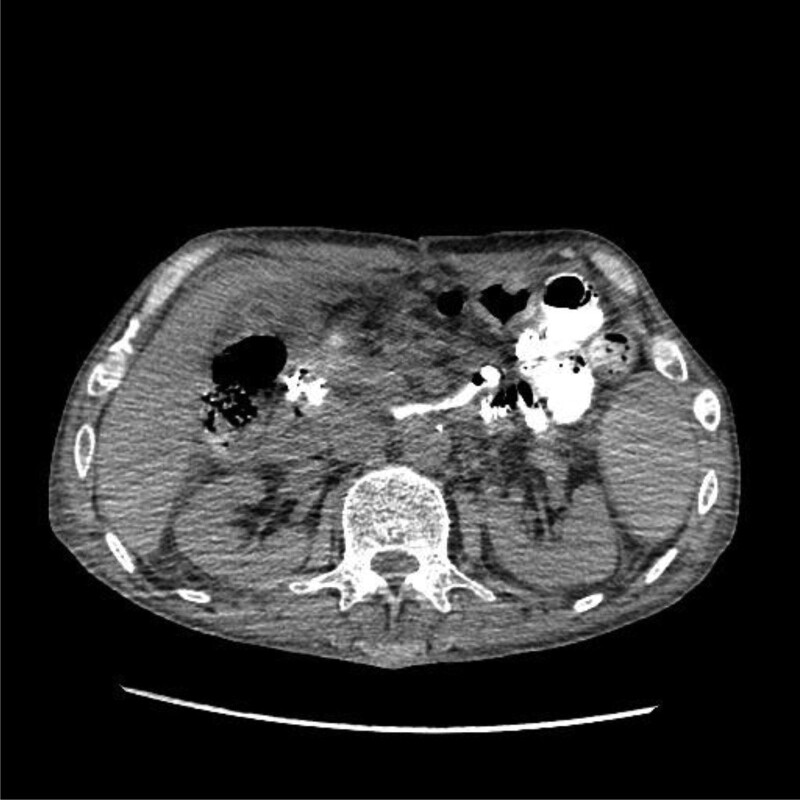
Abdominal computerized tomography (CT) examination was performed 15 minutes after oral contrast agent. Contrast agent can be seen in and around the peritoneal drainage tube, and duodenal leakage was considered.
